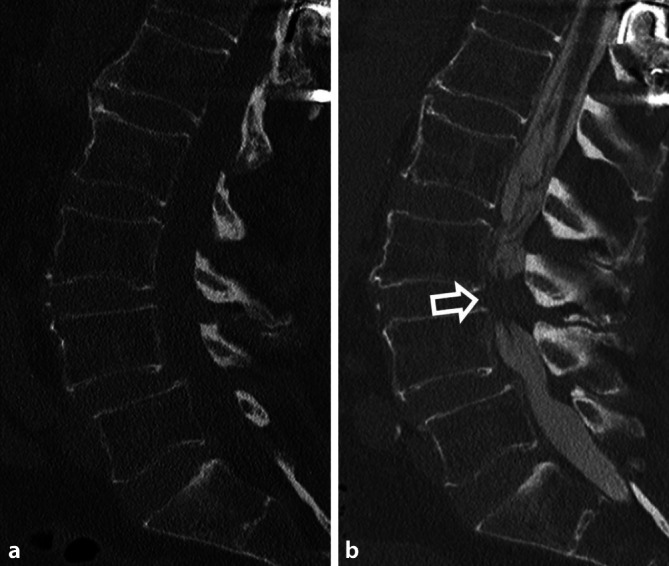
Die Notwendigkeit des Einsatzes von ionisierender Strahlung und eine erschwerte Beurteilung des Weichteilgewebes bleiben jedoch Nachteile der CT.
